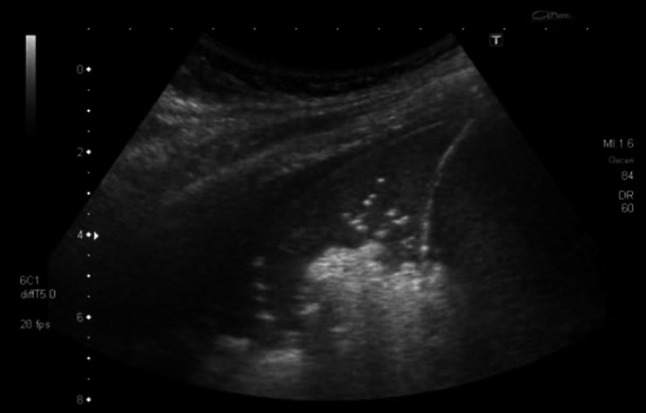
Transthoracic ultrasonography showing a hypoechoic consolidation with string-like echogenic reflexes due to remaining air in the bronchial system (sonographic air bronchogram). Additionally, a small pleural effusion is visible
